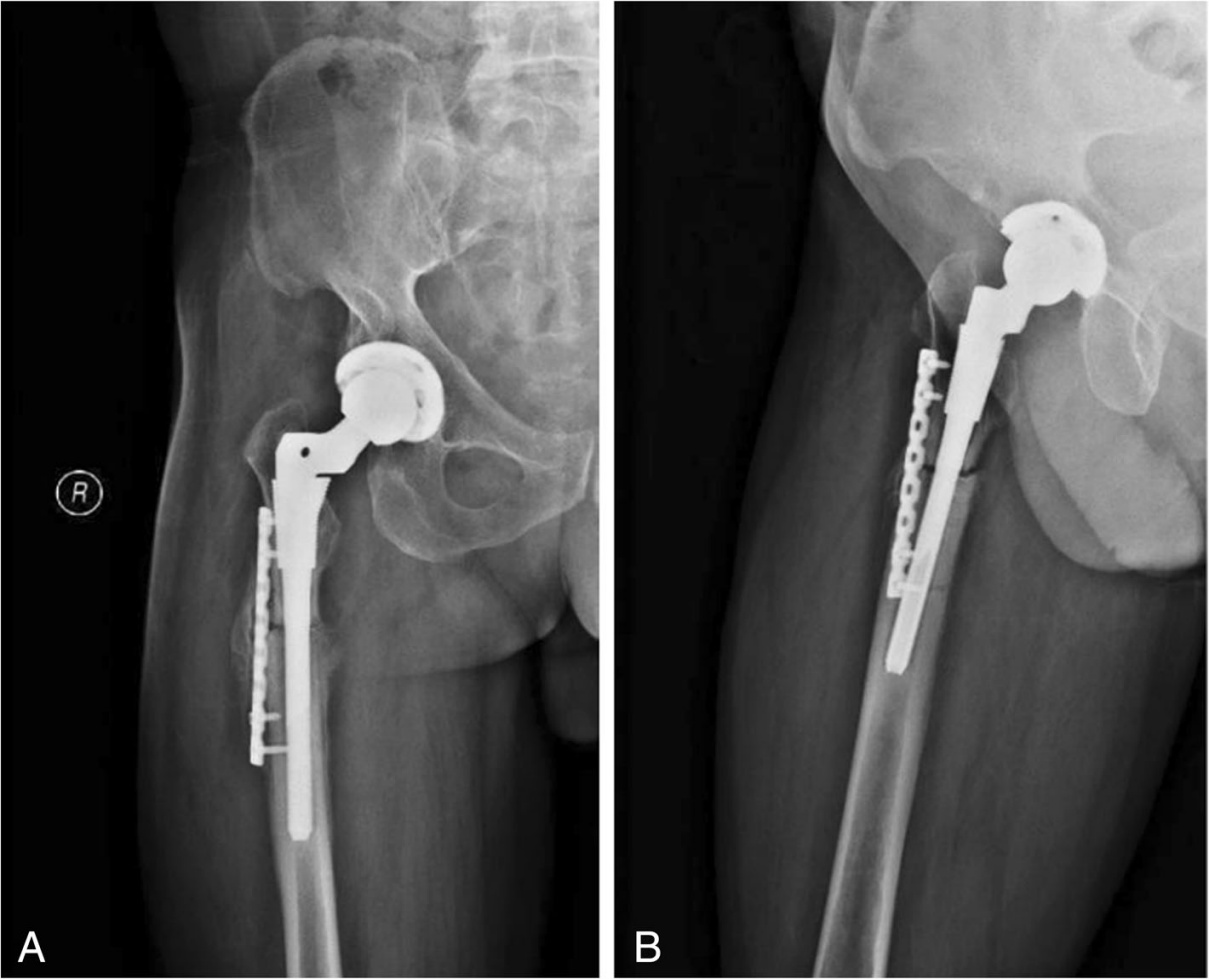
Radiographs of the right hip at 5 months after internal fixation was applied. (a) anteroposterior view of the right hip, (b) lateral view of the right hip
